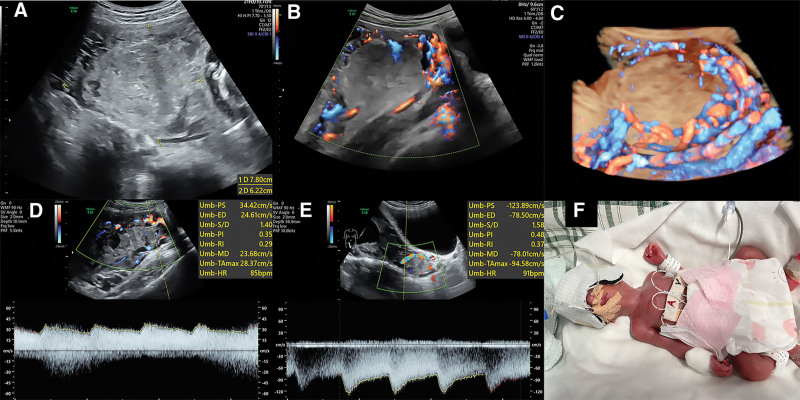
(A) The complex mass with solid and cystic components was on the right posterior uterine wall. (B) Multiple turbulent flows, consisting of a tangle of vessels with multidirectional high-velocity flow, were evident in and around the mass. (C) The three-dimensional color Doppler image of the mass showed hypervascularity in and around the lesion. (D) Spectral Doppler showed the low-resistance blood flow spectrum, the peak systolic velocity (PSV) was 34.42 cm/s, the resistive indexes (RI) was 0.29, and the pulsatility index (PI) was 0.35. (E) The uterine artery pulsatility index (UAPI) was 0.48 and RI was 0.37. (F) The newborn image of 26^+4^ weeks gestational age at birth. PSV = peak systolic velocity, PI = pulsatility index, RI = resistive indexes, UAPI = uterine artery pulsatility index.
